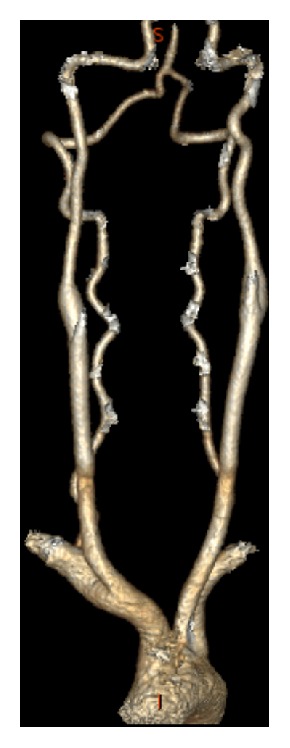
CT angiogram reconstruction of the neck shows no obstruction, dissection, stenosis, or abnormalities.
